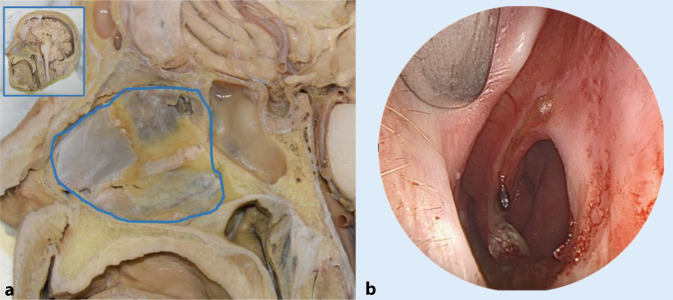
Ein NSD kann zu vielfältigen und unangenehmen Symptomen führen, wie eingeschränkte Atmung, Nasenbluten, trockene bzw. reflektorisch zu feuchte Nase sowie Schlafstörungen. Daher ist ein Verschluss des Septums angezeigt, um einen konstanten nasalen Luftstrom wiederherzustellen. Aktuell gibt es 2 therapeutische Strategien zur Behandlung von NSD-Symptomen: (1) den Einsatz von pflegenden Sprays und Salben zur Linderung der Beschwerden und (2) kausal der chirurgische bzw. nichtchirurgische Verschluss der Perforation. Die Perforation kann temporär mit einsetzbaren Nasenseptumbuttons oder operativ dauerhaft mit Eigengewebe verschlossen werden. Die Implantate werden anhand von analogen und digitalen Modellen gefertigt. Die Modelle werden entweder aus Silikonabformungen unter Vollnarkose oder in lokaler Betäubung oder aus computertomographischen(CT‑)Daten generiert. Beide Methoden haben jedoch einen Einfluss auf die Patientensicherheit, da sie das Morbiditätsrisiko erhöhen oder die Strahlenhygiene verletzen.
